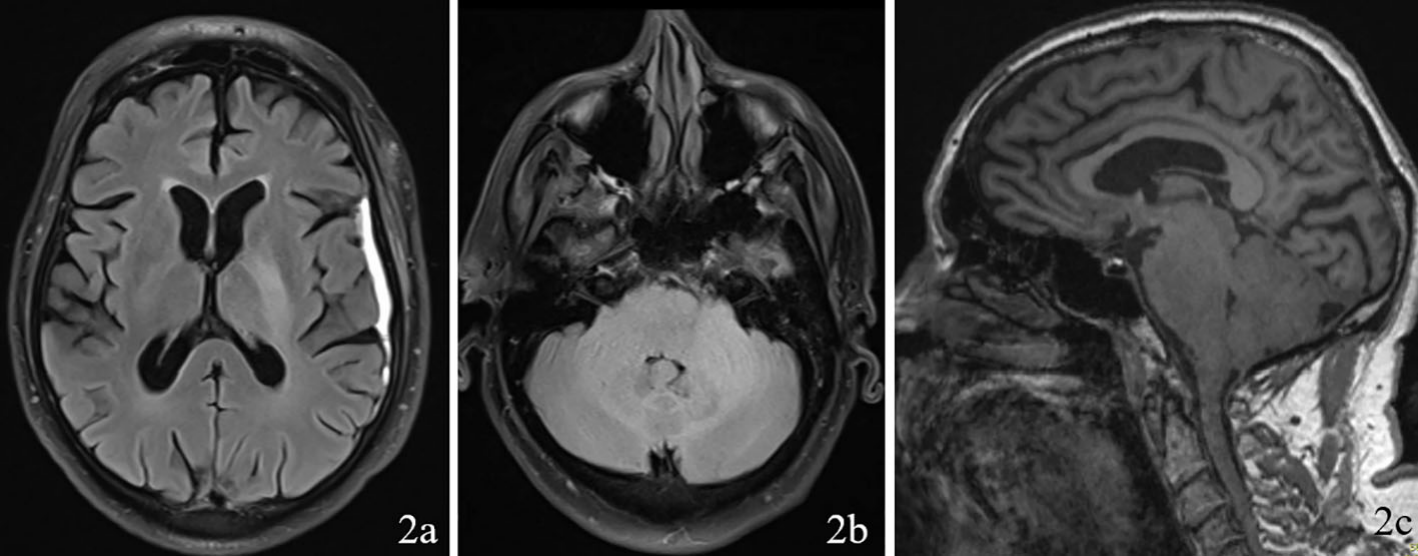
Imaging with FLAIR sequence on 2a (axial through internal capsule/corona radiata), 2b (axial through pons/cerebellum), and 2c (sagittal) demonstrating diffuse hypodensity of the left the pons and cerebellum.
